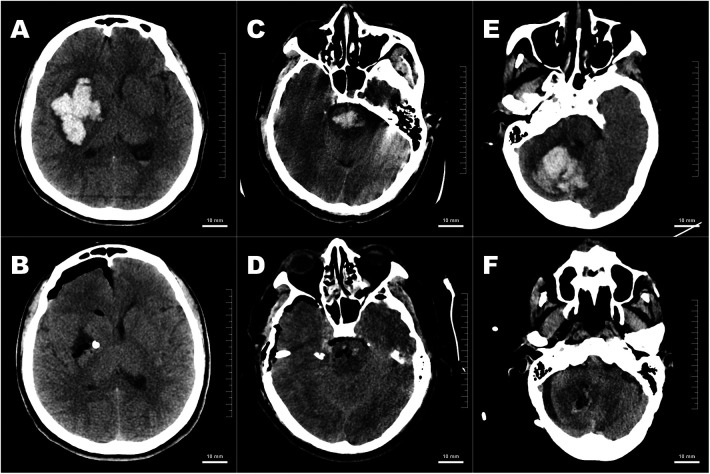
Pre- and postoperative axial CT images demonstrating hematoma evacuation across three hemorrhage locations. (A,B) Supratentorial (basal ganglia) hemorrhage: preoperative scan (A) shows a large hyperdense hematoma with a midline shift; postoperative scan (B) demonstrates near-complete clot evacuation and a reduction in the mass effect. (C,D) Brainstem (pontine) hemorrhage: Initial scan (C) reveals a dense central pontine hematoma; follow-up (D) shows a significant reduction in hematoma volume and an improved contour of adjacent structures. (E,F) Cerebellar hemorrhage: Preoperative image (E) indicating a space-occupying hematoma compressing the fourth ventricle; postoperative image (F) showing effective clot removal and decompression of posterior fossa contents.
